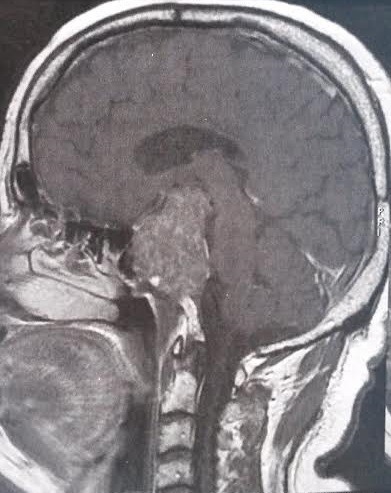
MRI of the brain showing an expansive intrasellar process of 3.7cm
